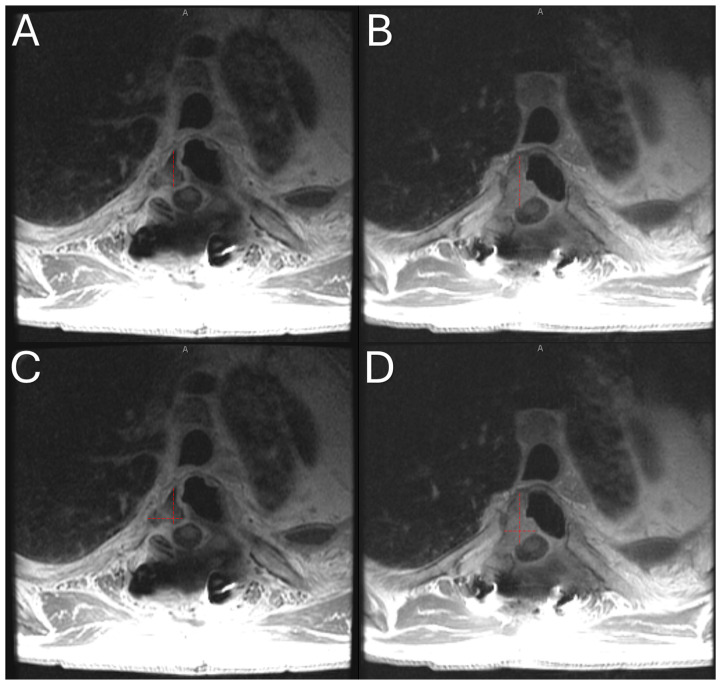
Representative case showing the unidimensional (RECIST1.1 (A,B)) and bidimensional (MDACC (C,D)) measurements for a locally progressive metastatic spinal lesion in an axial T1W-MRI at the T4 level, initial (A,C) and progression (B,D).
